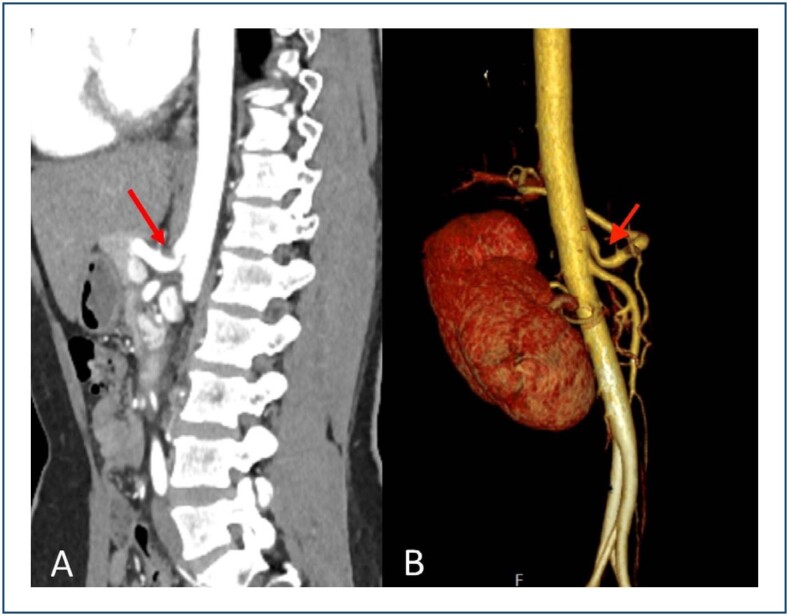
(A) Sagittal arterial phase computed tomography angiography image and (B) volume-rendered 3D reconstruction from abdominal aortogram. The hooked appearance of the proximal celiac axis (red arrow) with poststenotic dilatation is being caused by the median arcuate ligament indenting upon the superior aspect of the artery.
